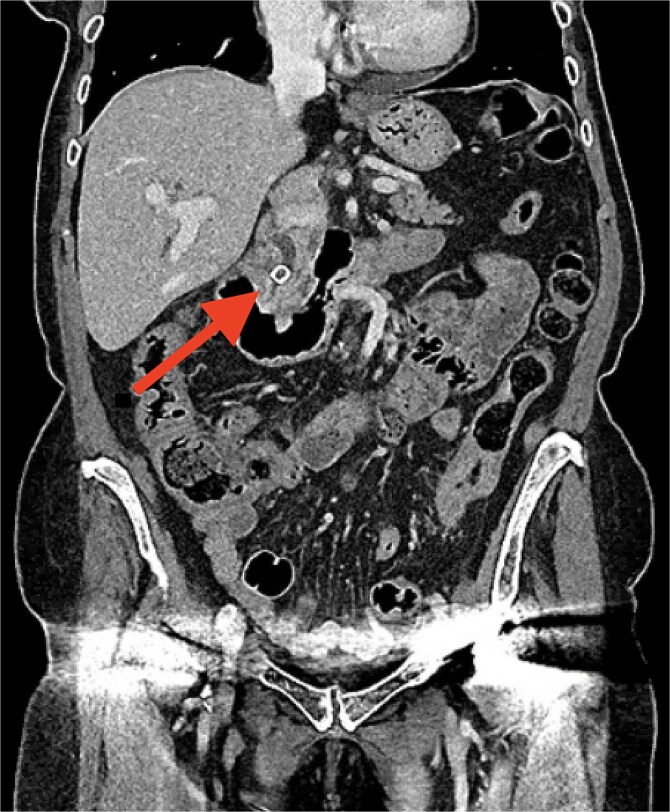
Initial CT demonstrating the obstructing distal common bile duct stone. *This image was obtained at University of Oxford Hospitals NHS Foundation Trust with patient consent.*
